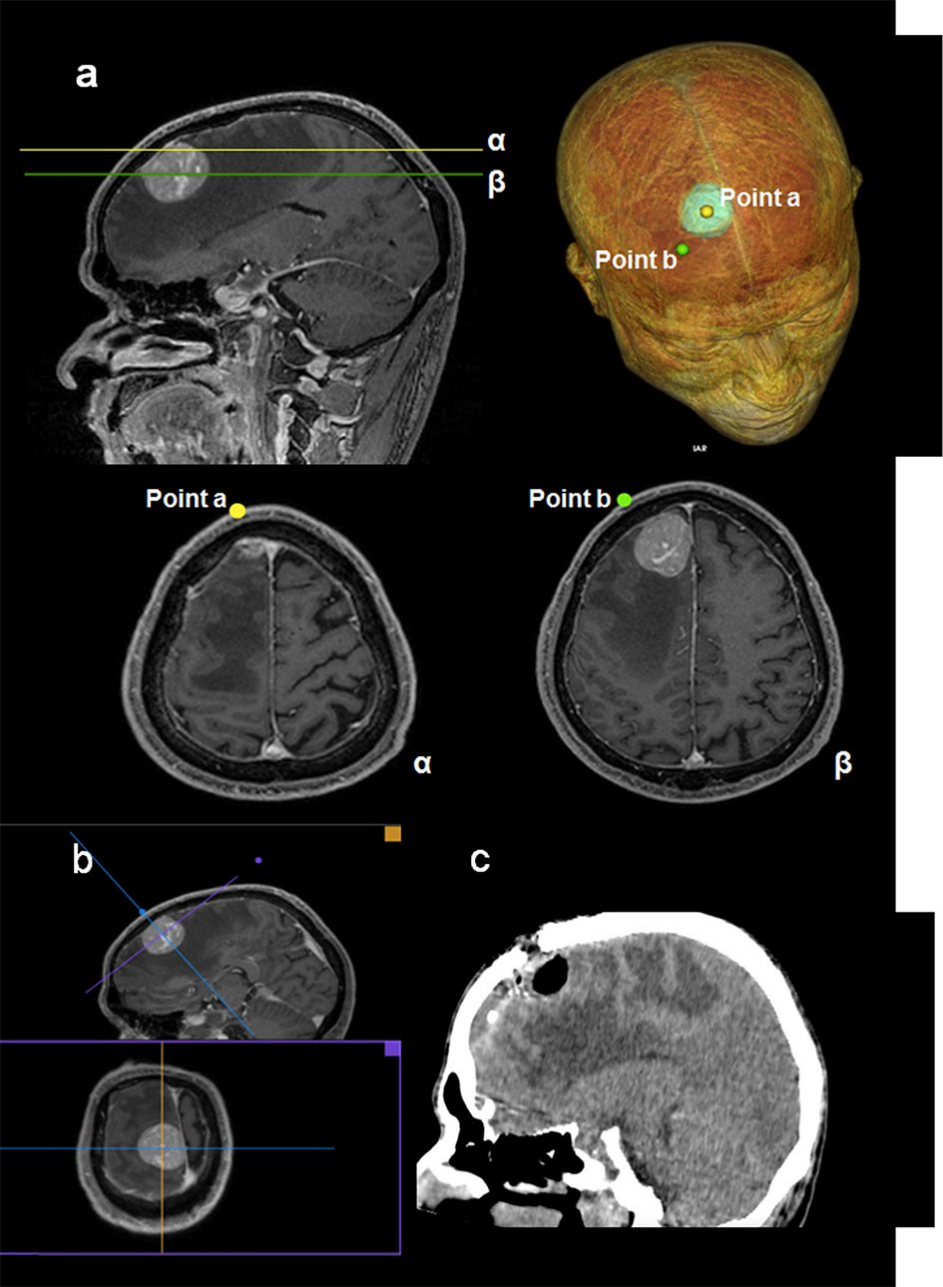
Demonstration of the importance of multiplanar reconstruction in a case of superior frontal gyrus tumor. A 58-year-old man presented to the hospital because of left hemiparesis. (a) Cranial MR image demonstrating two slices (α and β) with two options of target points (a, b), as well as 3DVR showing the points and the tumor; (b) multiplanar reconstruction perpendicular to the intended approach axis; (c) postoperative cranial CT scan demonstrating tumor gross total resection. A linear right frontal incision and a right frontal minicraniotomy were performed and the patient evolved with neurological improvement.
